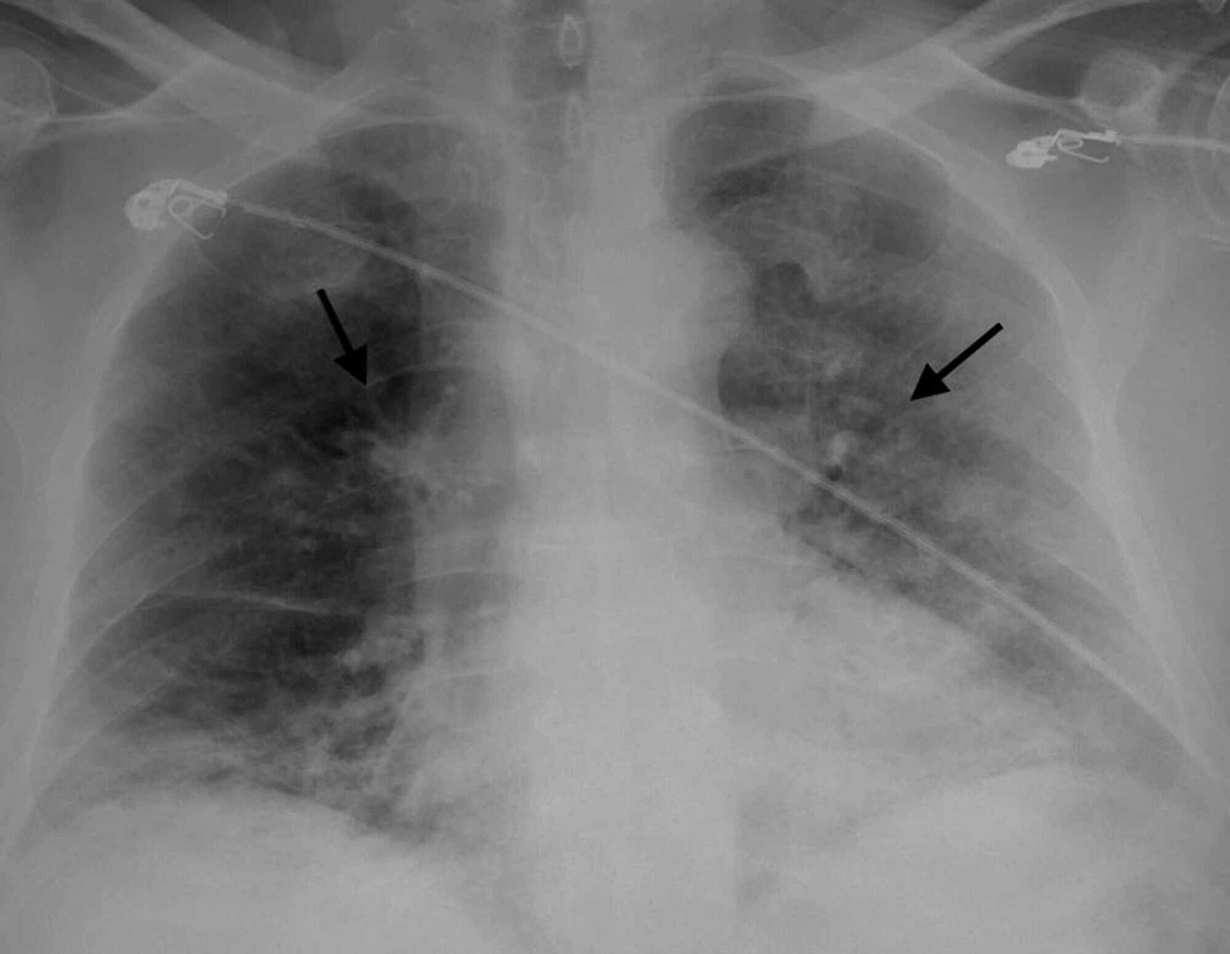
Chest X-ray shows bilateral infiltrates as shown by arrows
